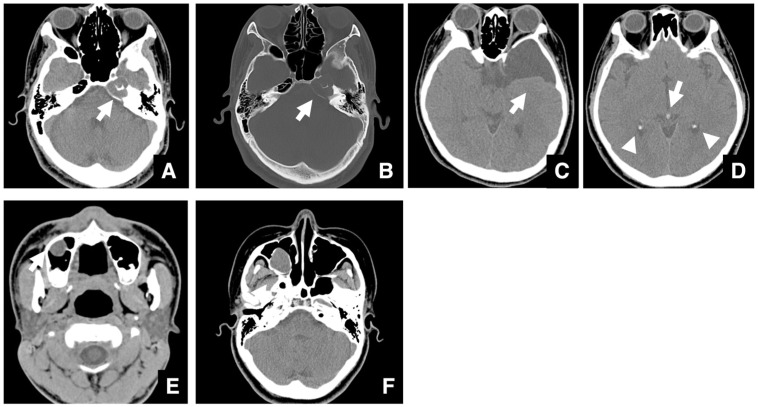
Representative multidetector (MD) row CT images (slice thickness, 3.0 mm) of incidental findings detected during evaluation of jaw deformities. (A) Soft tissue and (B) bone window images of a patient with a brain tumor (arrow) located in the left petrous region. (C) Soft tissue CT image of a patient with an arachnoid cyst (arrow) located anterolaterally on the left. (D) Soft tissue CT image of a patient shows calcification of the pineal gland (arrow) and choroid plexus (arrowheads). Soft tissue CT image of a patient with a retention cyst (arrow) in the maxillary sinus. (E) The lesion shows lower density than muscle and is not surrounded by bone. Soft tissue CT image of a patient with a dentigerous cyst (arrow) expanding into the maxillary sinus. (F) The lesion is surrounded by a thin layer of bone. Permission has been obtained to publish all figures.
